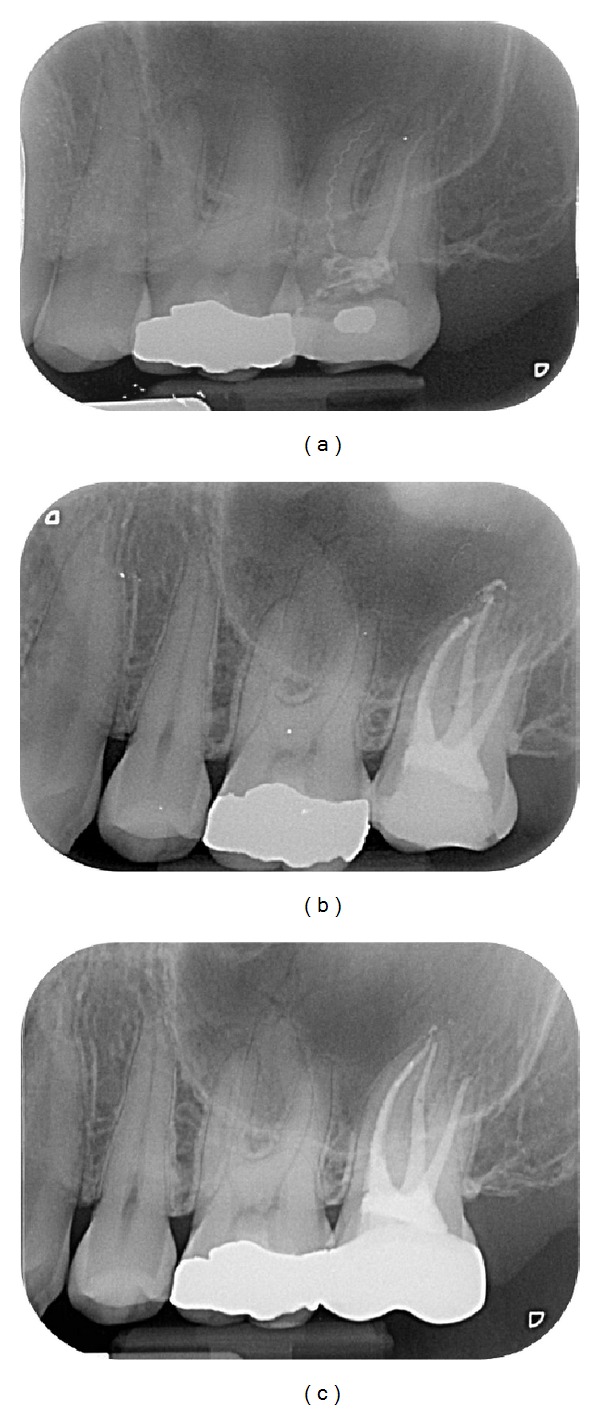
Successful endodontic retreatment of 27 symptomatic, with broken instrument. (a) Preoperative X-ray of a patient sent for orthograde retreatment of 27, painful, with broken instrument (Lentulo) in the mesio-buccal root. The initial treatment was recent. (b) Peroperative X-ray: the broken instrument has been removed and apical patency was found before the cleaning, shaping, and filling of the canal. (c) Recall X-Ray with follow-up for 4 years. Note that lateral radiolucency had disappeared. The tooth is classified as “healed”: absence of symptoms and periodontal healing.
